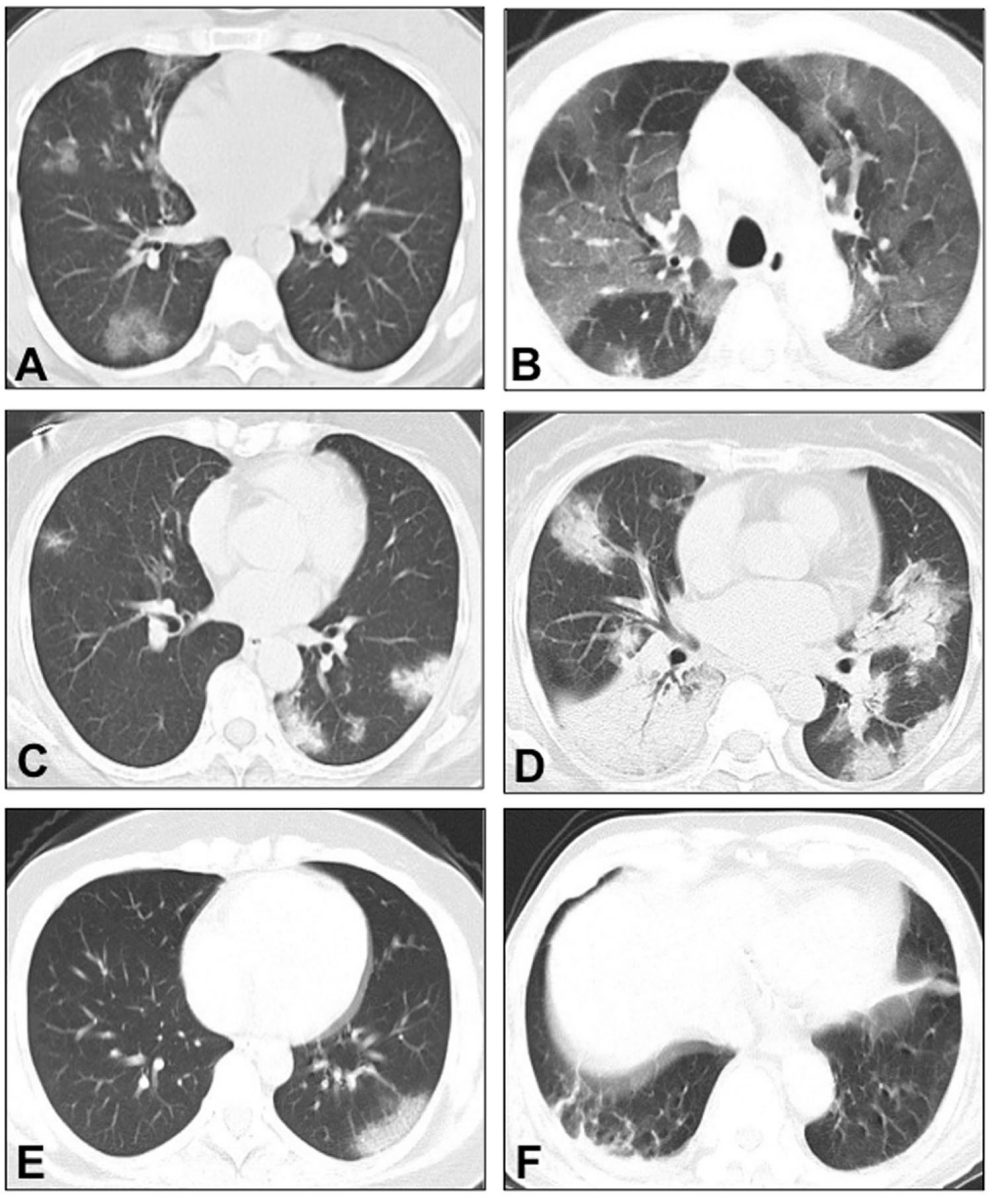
Radiographic features of HRCT scans in patients with confirmed COVID-19. Representative HRCT images showing (A) ground glass opacity in a 60-year-old man with pneumonia; (B) ground glass opacity and air bronchogram in a 65-year-old man with severe pneumonia; (C) consolidation in a 56-year-old woman with pneumonia; (D) consolidation and air bronchogram in a 57-year-old woman with severe pneumonia; (E) nodular opacities in a 24-year-old woman with pneumonia; (F) pleural effusion of the right chest in a 70-year-old man with severe pneumonia.
